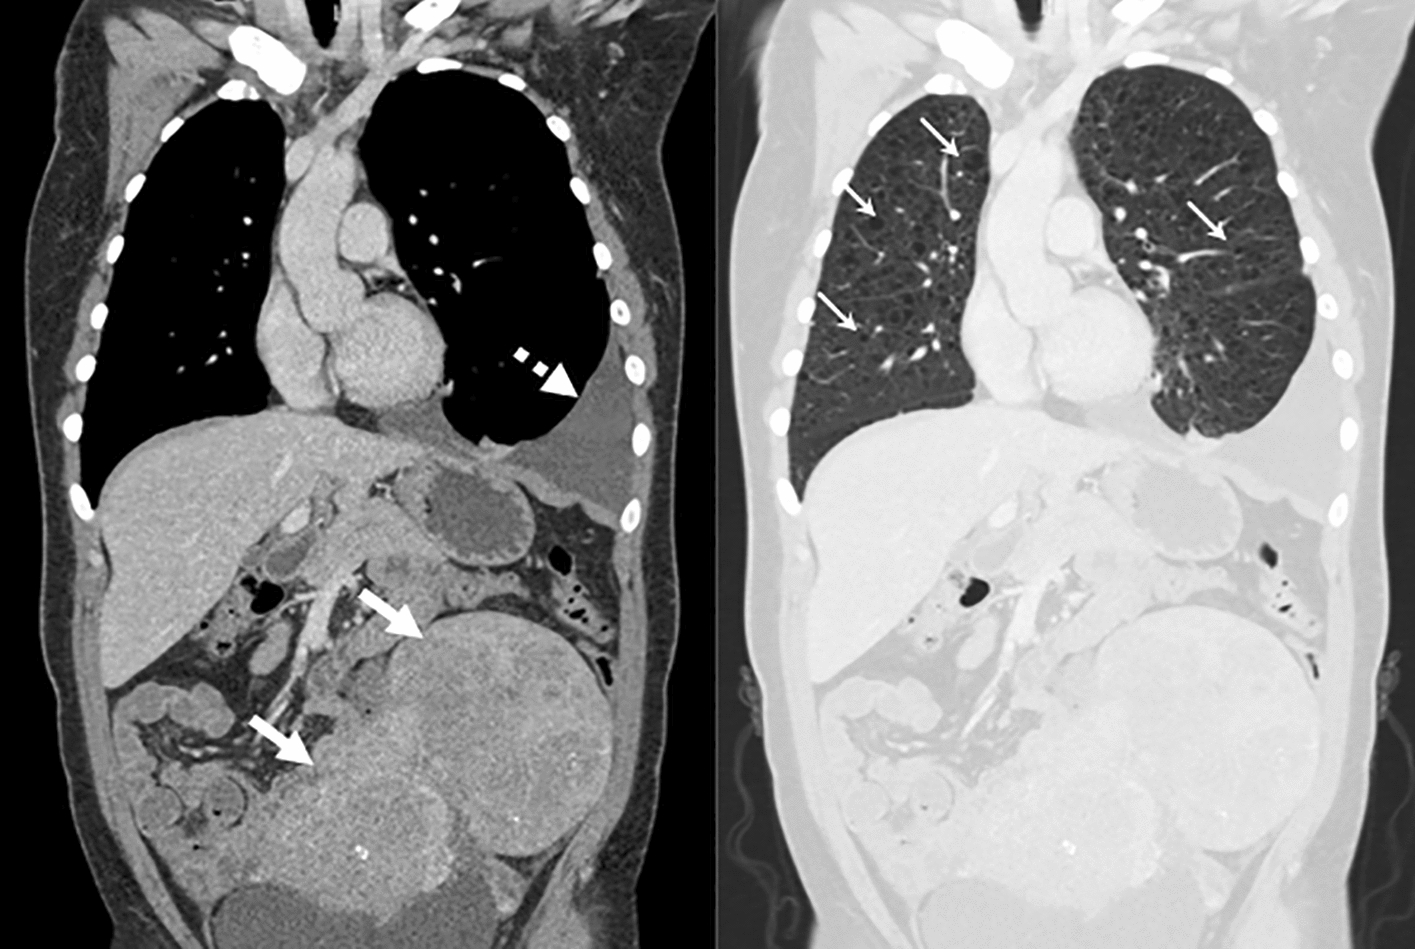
Clinical manifestations of LAM. Coronal CT scan of the chest and abdomen of a patient with LAM. Soft tissue windows (left) show a large, complex abdominal lymphangioleiomyoma (white arrows) and a left sided chylous pleural effusion (dashed arrow). Lung windows (right) show multiple air-filled lung cysts distributed throughout the lung parenchyma (some highlighted by small arrows)
